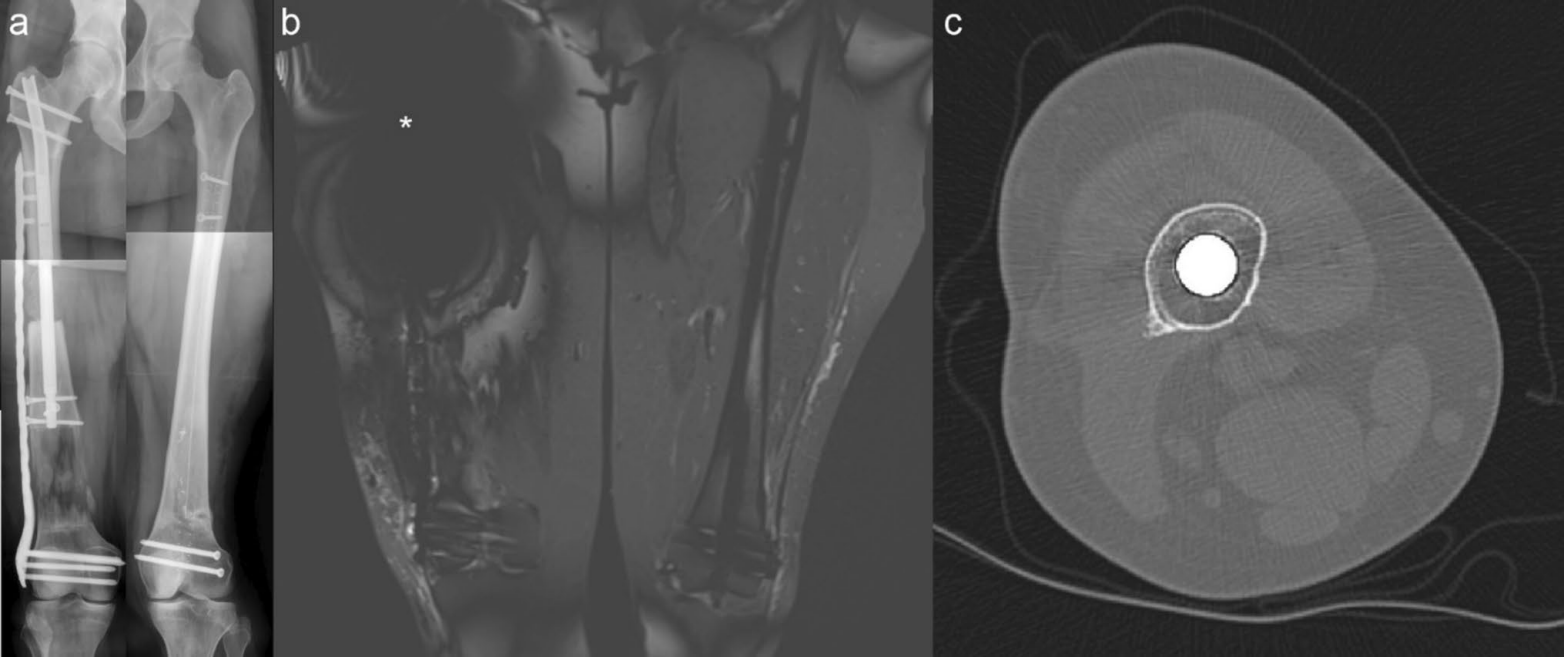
Anteroposterior radiographs of the bilateral femurs demonstrate the right femur undergoing plate-assisted bone segment transport with a titanium plate (Stryker Corp., Kalamazoo MI, USA) and fixation of the left femur with a carbon fiber nail (CarboFix Orthopedics Ltd., Herzeliya, Israel) (a). A coronal STIR MRI of the bilateral femurs demonstrates significant artifact surrounding the internal magnet of the Precice nail (*) compared to the plate and carbon fiber nail (b). An axial CT of the femur directly through the internal magnet of the Precice nail demonstrates minimal artifact with adequate visualization of the surrounding tissues (c)
